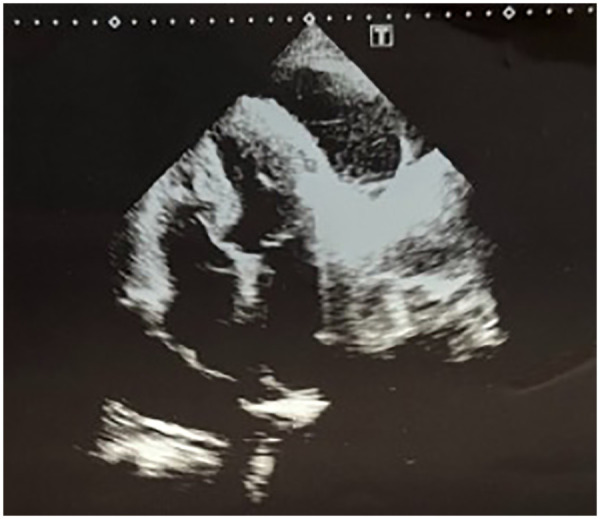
Echocardiogram image displays a fourchamber view of the heart showing detailed anatomical structures including ventricles and atria, with grayscale contrast representing tissue density and blood flow.
